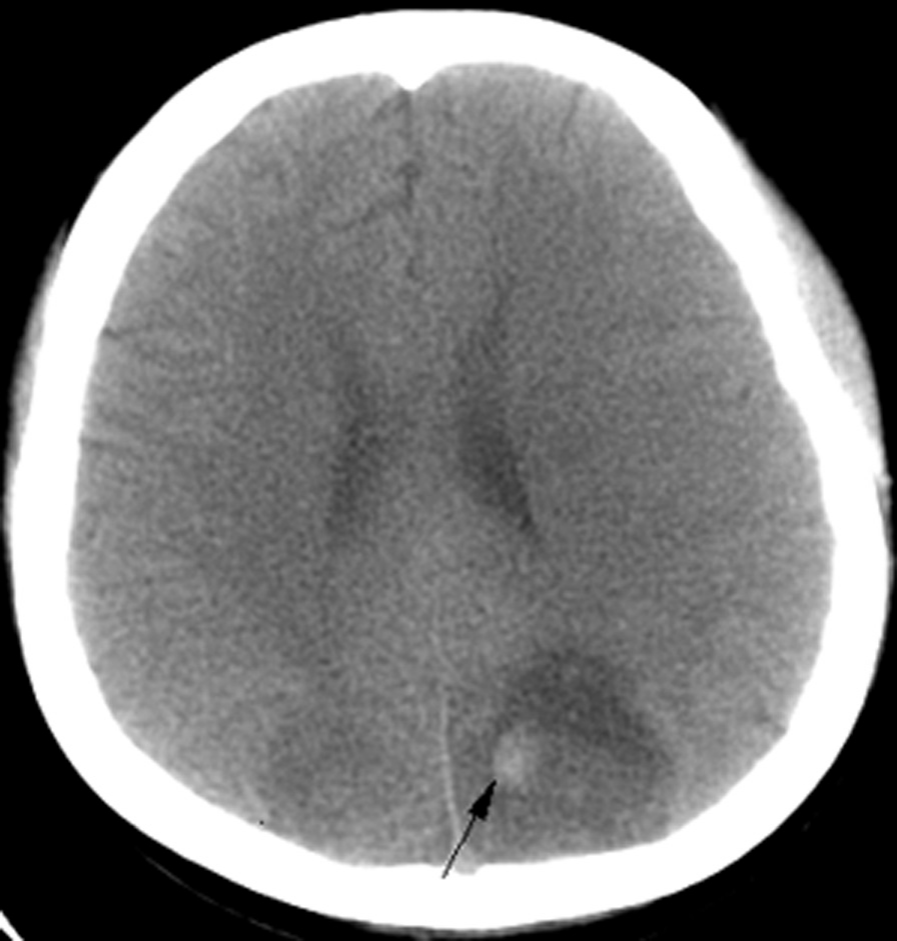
CT of a 19 year-old SLE patient who presented with coma. CT image on brain show showing bilateral hypodensities involving gray and white matter in posterior circulation territories as well as a right occipital hematoma (arrow).
